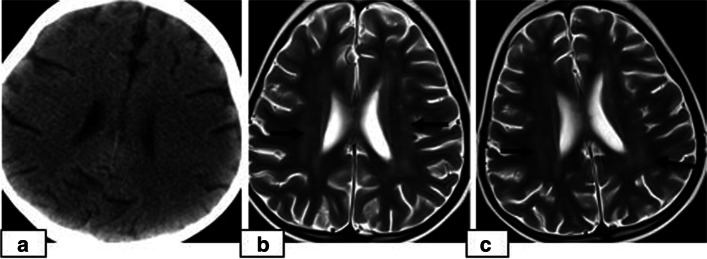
An 8-year-old girl presenting with headache, nausea and vomiting 5 months after undergoing allogeneic HSCT for ALL. a Axial CT of the head did not reveal any abnormalities. b Axial T2W image showing increased signal intensity in the periventricular white matter (b, arrows). d Follow-up axial T2W image 3 months after the first presentation shows persistent increased signal intensity in the periventricular white matter (c, arrows)
